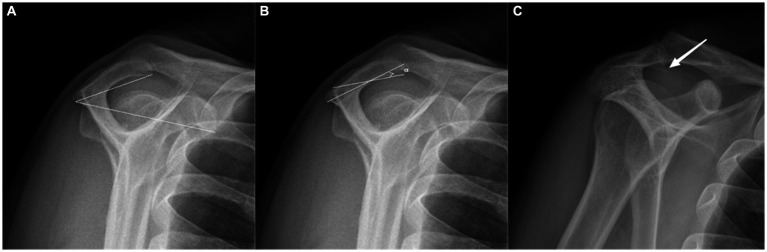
Radiographic measurements on the outlet-view radiograph. (A) Acromial tilt (AT), a type II (curved) acromion according to the Bigliani. (B) Acromial slope (AS). (C) Acromial spur.
